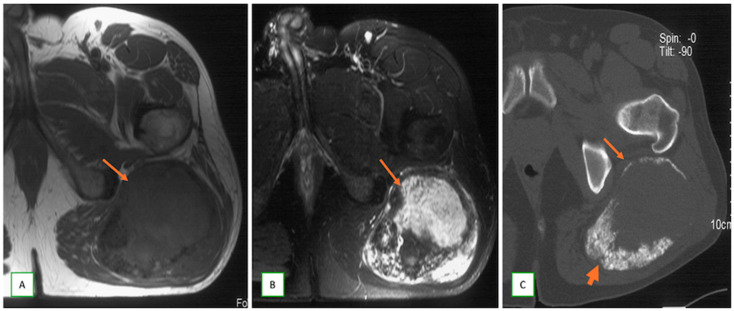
Malignant peripheral nerve sheath tumor (MPNST) of the sciatic nerve in a 51-year old man presenting with left buttock swelling. Axial T1 (A), axial fat saturated T2 (B), and axial CT (C) images showing the large, heterogeneous mass (thin arrows) with low to intermediate T1 signal, high T2 signal with central necrosis/hemorrhage, and peripheral calcification (thick arrows) on CT.
